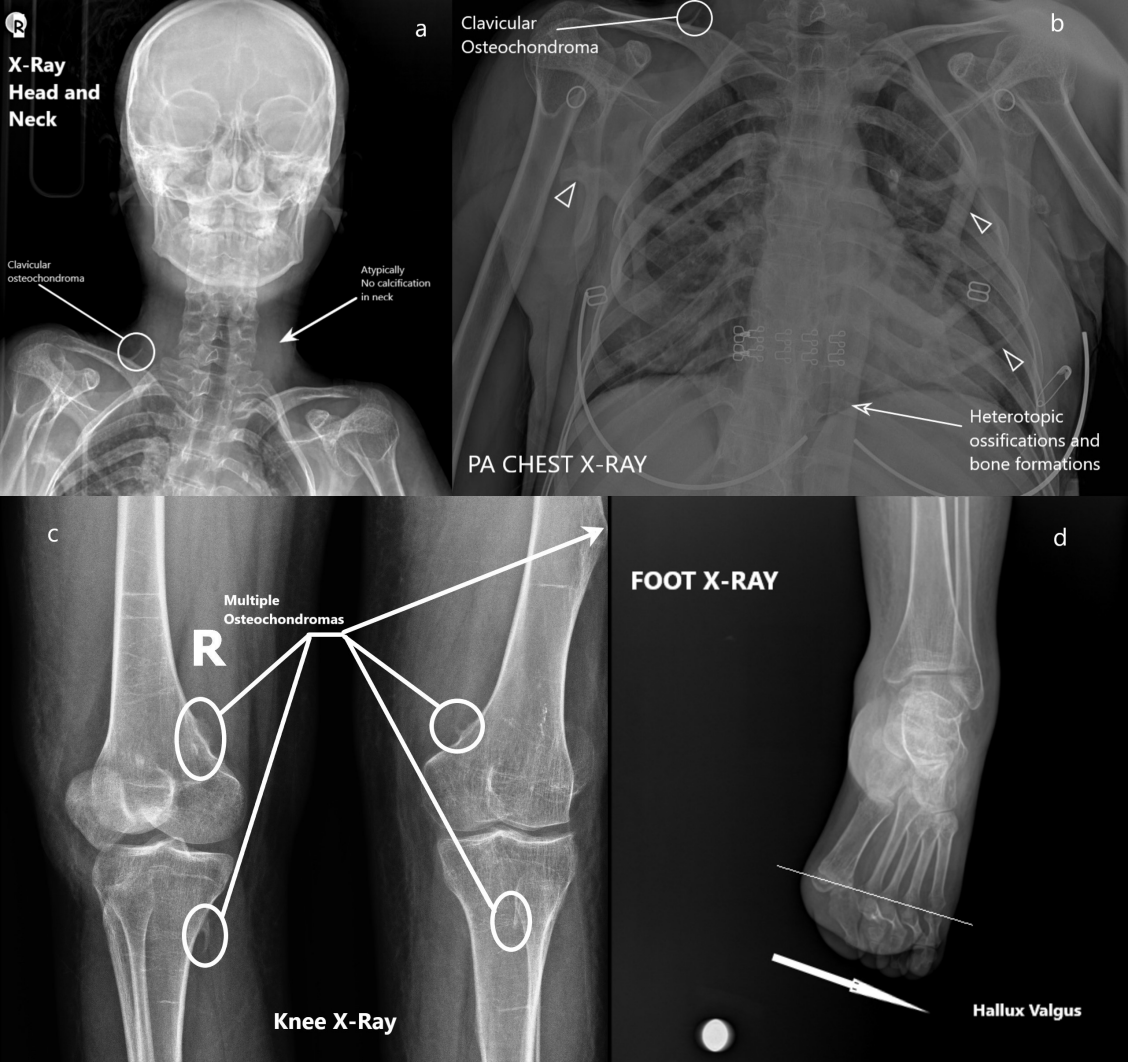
X-ray graphs; a) atypically no calcifications or ossifications in the neck, right clavicular osteochondroma; b) heterotopic ossifications and bone formations around the chest (arrowhead), compact bone formations along with vertebral axis, clavicular osteochondroma; c) multiple osteochondromas located around the knee joint; d) hallux valgus anomal
